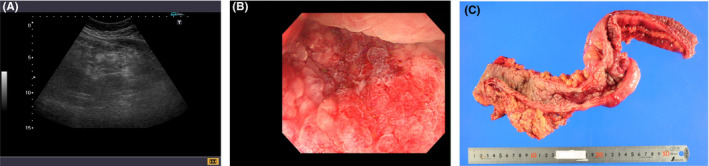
A, Ultrasonography detecting a hypoechoic portion with a central hyperechoic region, confirming a pseudokidney sign. B, Colonoscopy showing circumferential long ascending colon stenosis with cobblestones nodularity. C, The tumor extending till the ascending colon, Bauhin's valve, appendix, and terminal ileum without intussusception
